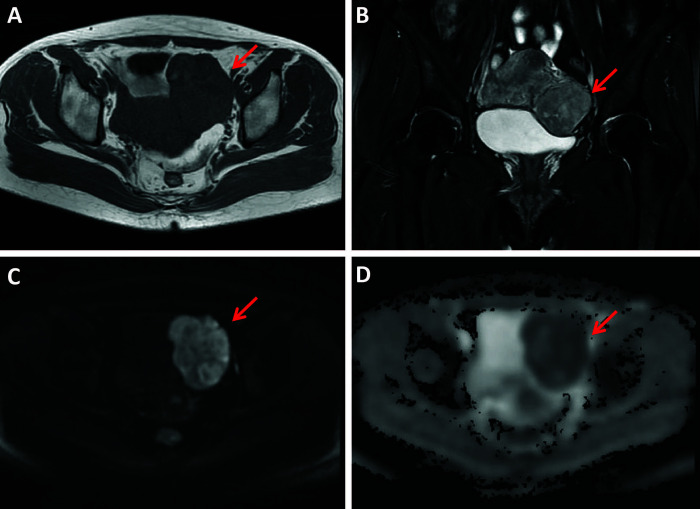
A 49-year-old woman with a uterine sarcoma. A mass was found in the uterine-side wall (arrow) with an unclear margin, showing heterogeneous iso-to-hyperintensity on T1WI (A) and heterogeneous hyperintensity on T2WI images (B). The tumor showed high signal intensity on diffusion-weighted MR image (C) with a low ADC value (ADC = 736 × 10^-6^ mm^2^/sec) (D).
